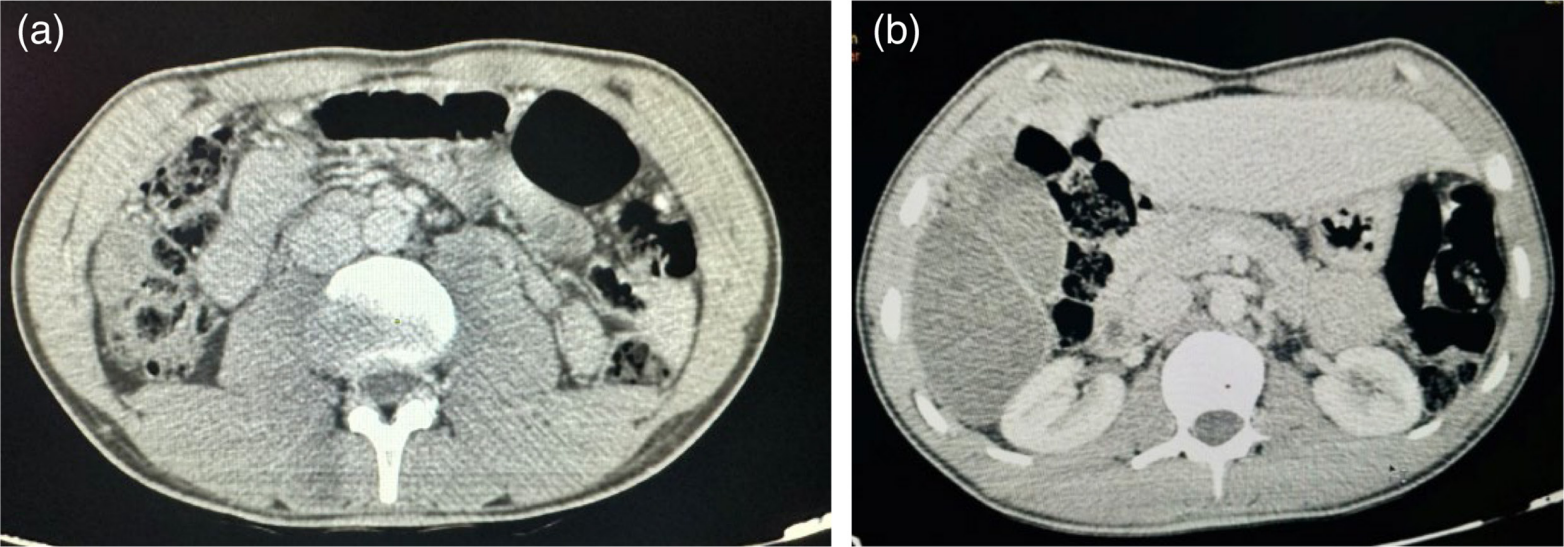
Abdominal CT scan (cross-section) showing a large hydatid cyst in the right lobe of the liver with peritoneal dissemination in the inter-hepato-phrenic space and along the Arantius groove.
